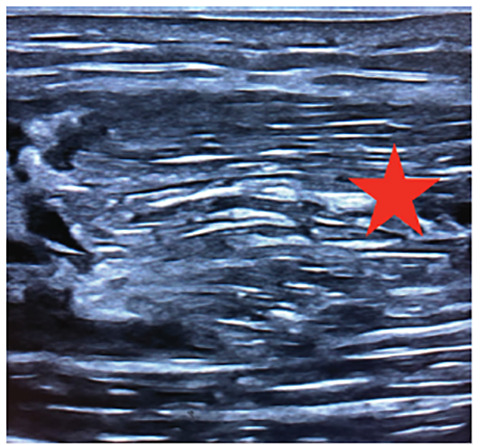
Point-of-care ultrasound in the longitudinal view of the mid long head biceps tendon (LHBT). The star indicates the fluid-filled area where the LHBT should be located with small, echogenic blood clots throughout, indicating a tendon rupture.
